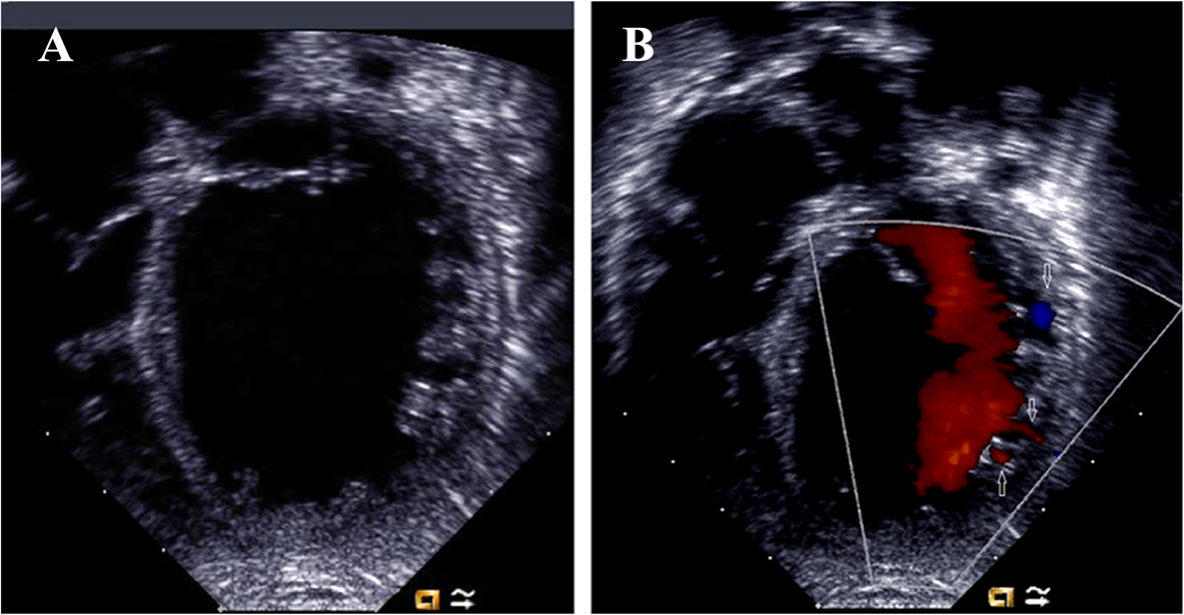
Echocardiogram (apical four-chamber view) of patient BTHS5 depicting LVNC with associated DCM phenotype. a Two-dimensional echocardiogram demonstrating the two-layer structure of noncompacted and compacted layers. b Color Doppler echocardiogram demonstrating flow within deep intertrabecular recesses (*arrow*) in continuity with the left ventricular cavity. LVNC = left ventricular noncompaction; DCM = dilated cardiomyopathy
